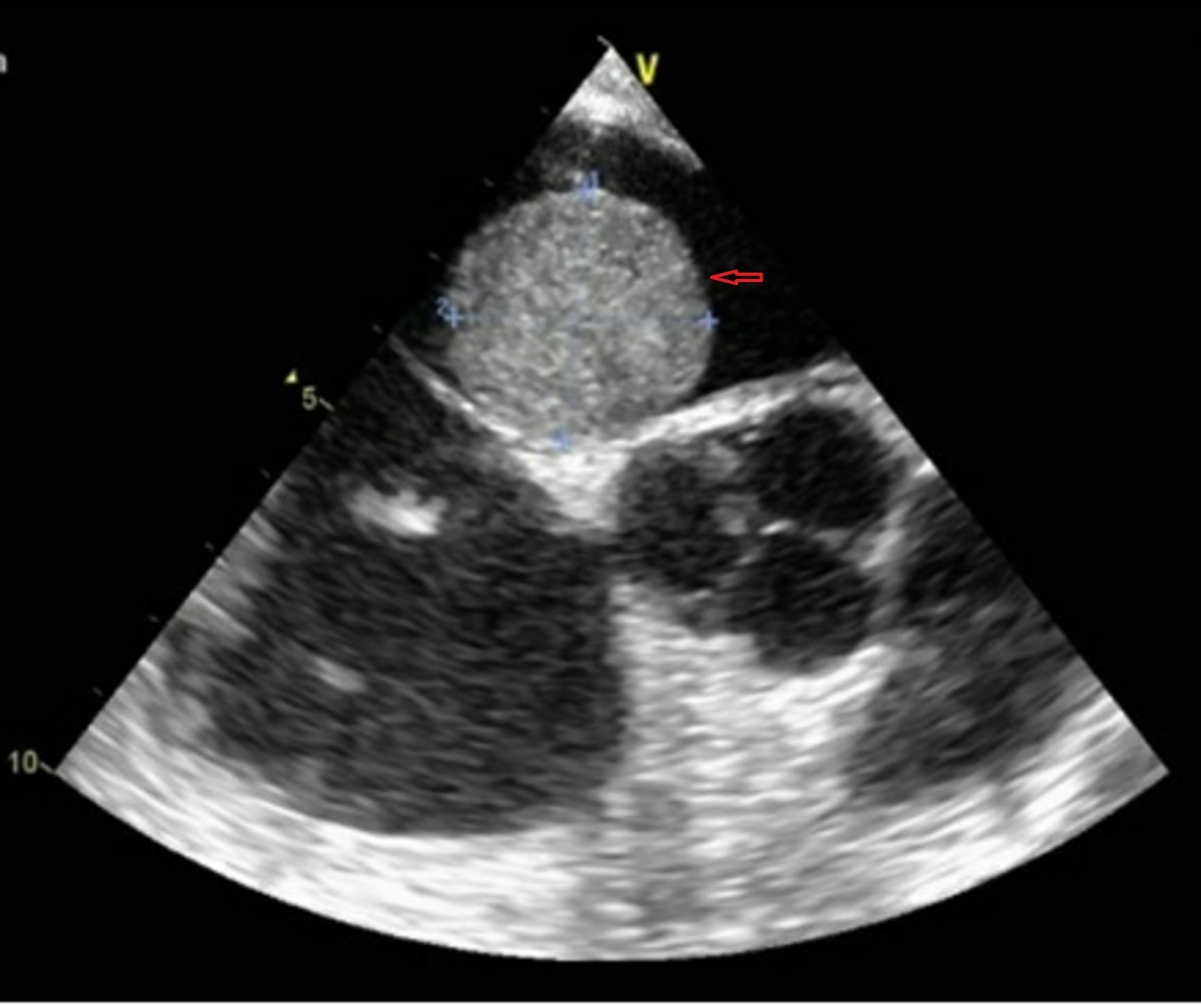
TEE frame shows left atrial myxoma. Red arrow points to left atrial myxoma. TEE: Trans-esophageal echocardiogram.
